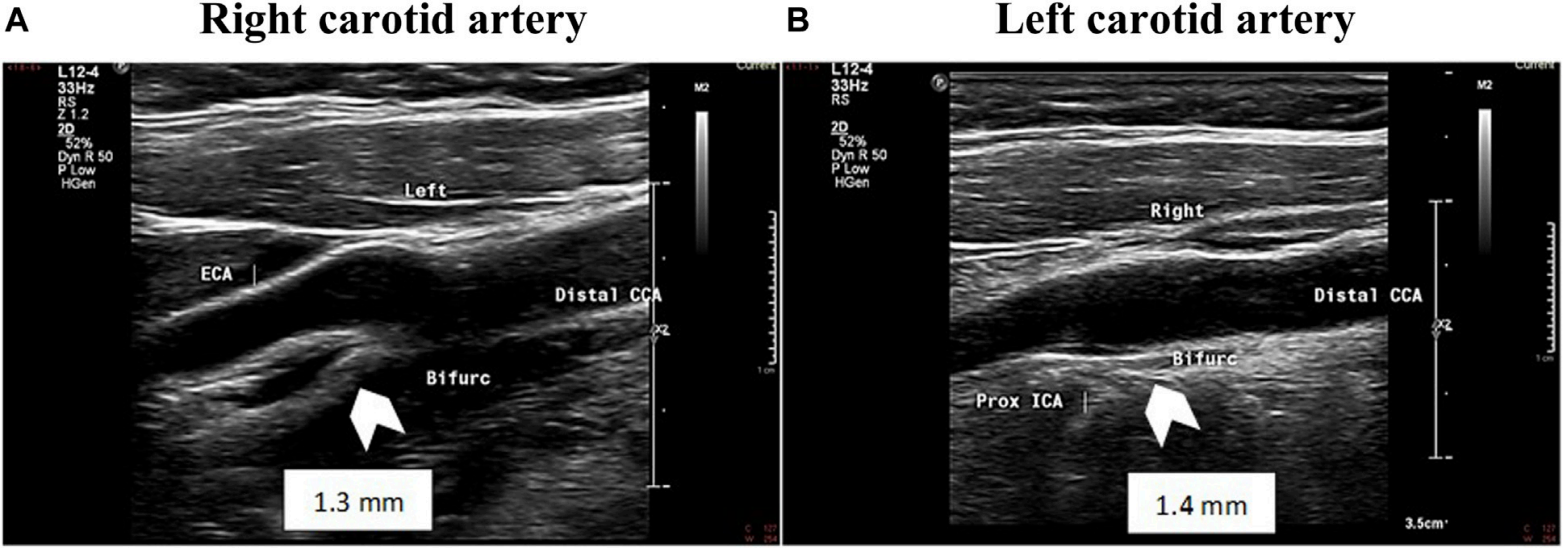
Carotid Intima media thickness. Ultrasound image showing measurement of near and far wall IMT in the distal 1 cm of the common carotid artery and increased carotid IMT of the common carotid artery (1.3 mm; 1.4 mm) of patient 2. White arrowhead -indicating increased carotid IMT. CCA, common carotid artery, ICA-internal carotid artery, Bifurc-bifurcation, mm-millimeter. (A) Right carotid artery, (B) B .Left carotid artery.
